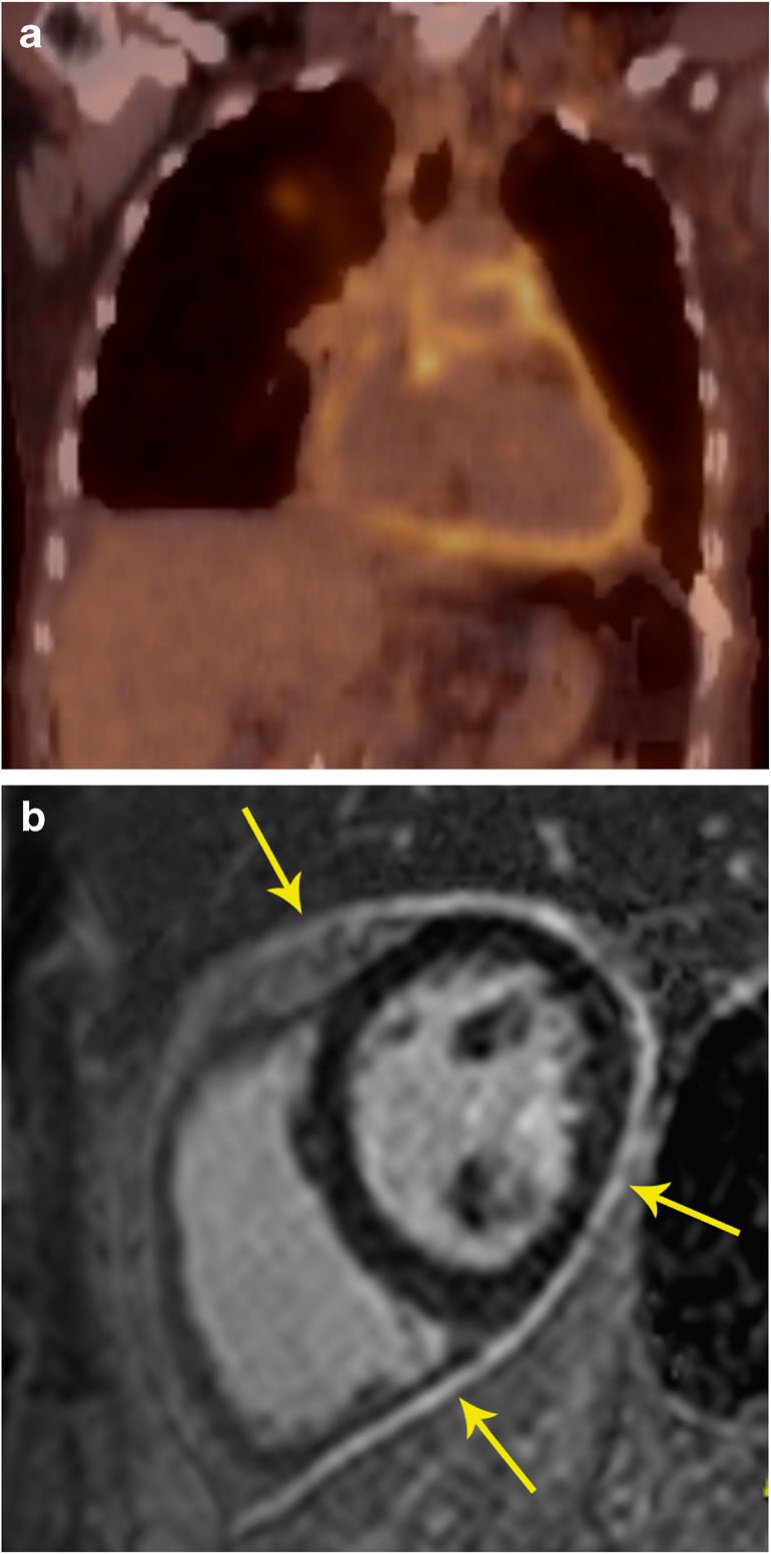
Pericarditis. a Coronal FDG PET/CT in a patient showed intense uptake in the pericardium. b Short-axis delayed enhancement MRI shows diffuse circumferential delayed pericardial enhancement (arrows), which is consistent with pericardial inflammation. There was also pericardial thickening in black blood images (not shown here)
